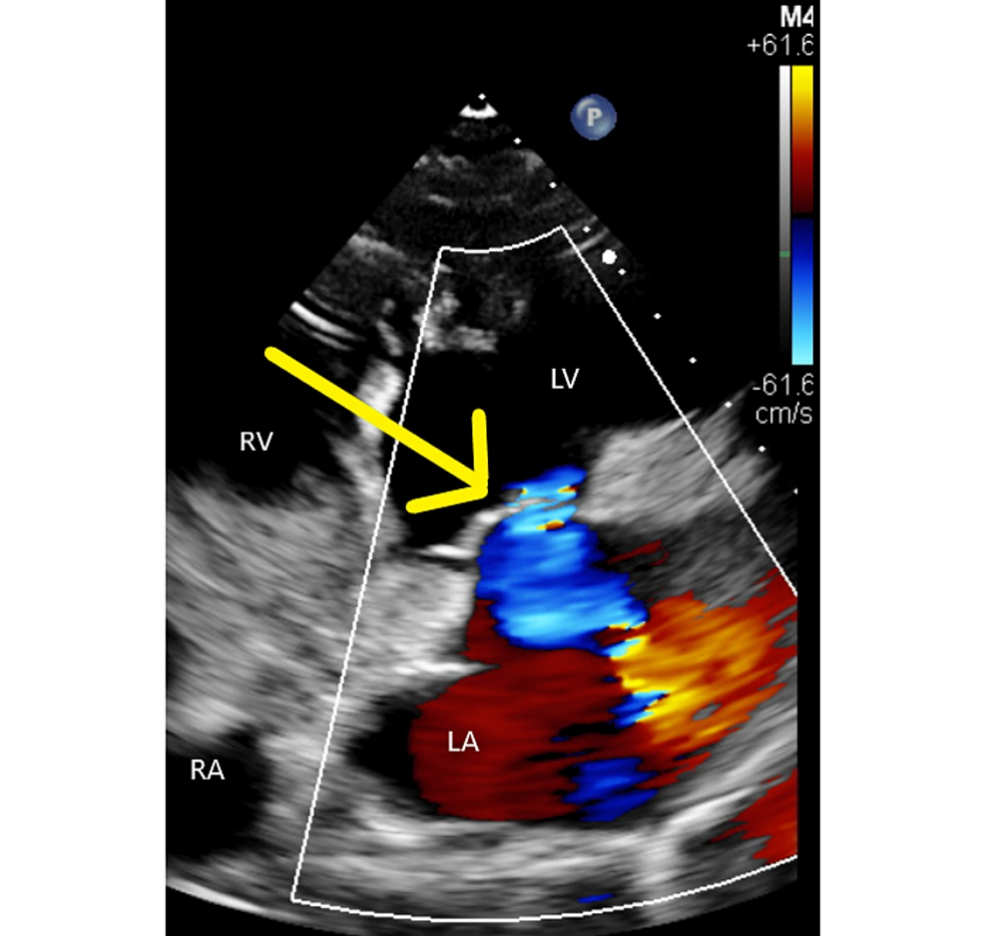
Parasternal view of transthoracic echocardiogram The yellow arrow points to the prosthetic mitral valve. LA: Left Atrium. The left atrium appears dilated with the mixed colors indicating regurgitant blood flow from the left ventricle to the left atrium through the prosthetic mitral valve. LV: Left ventricle, RV: Right ventricle, RA: Right atrium
